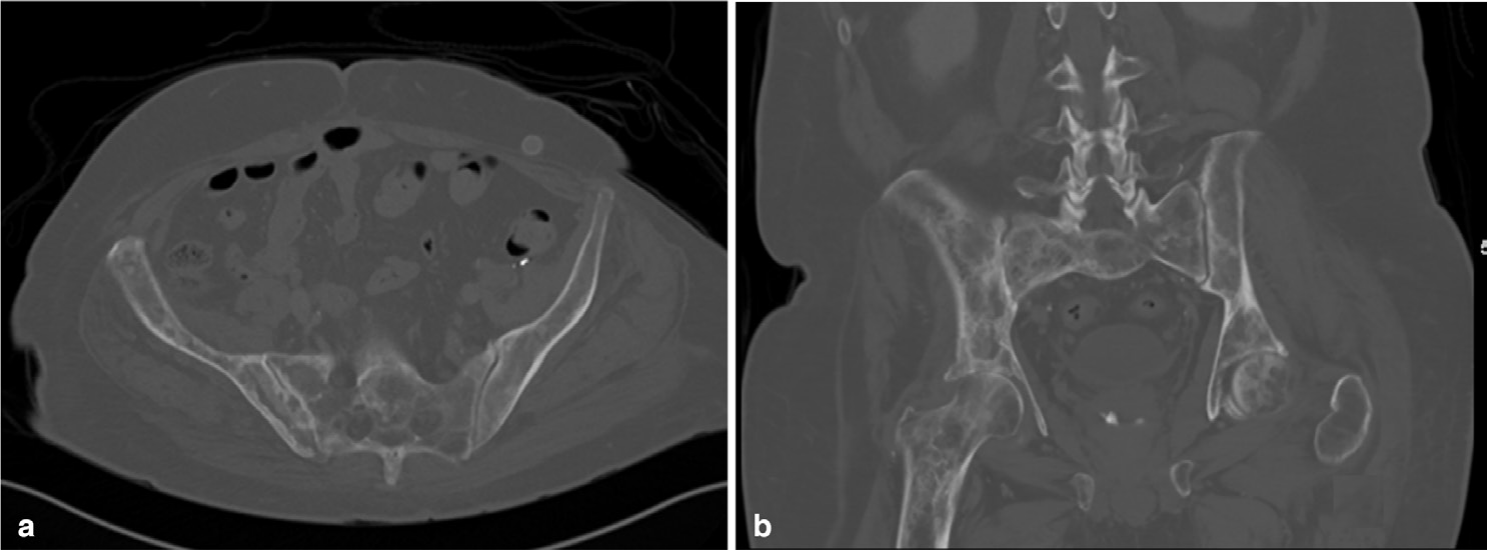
Axial (a) and coronal (b) follow-up CT scan at bone window about 15 months after initial presentation shows resolution of the pelvic and femoral intraosseous gas; however, signs of chronic bony osteonecrotic changes can be visualised.
